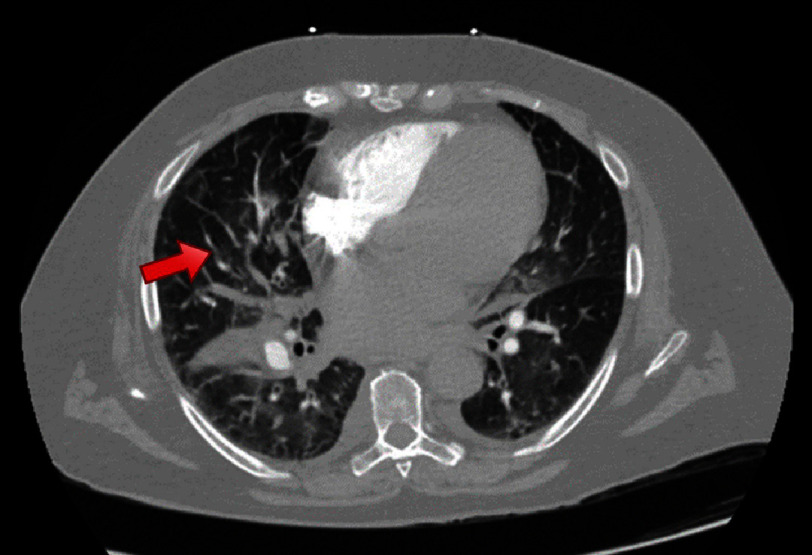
CT showing segmental pulmonary embolism (red arrow) within the anterior pulmonary artery branch to the right upper lobe.
